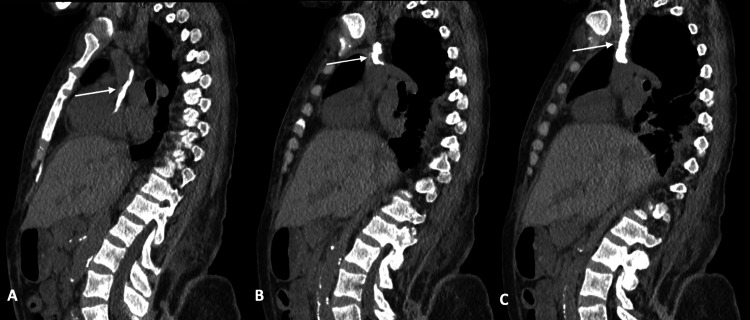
Noncontrast sagittal CT images from left lateral to right (A-C). Images demonstrate a right internal jugular vein calcified cast with its distal tip in the right atrium.
